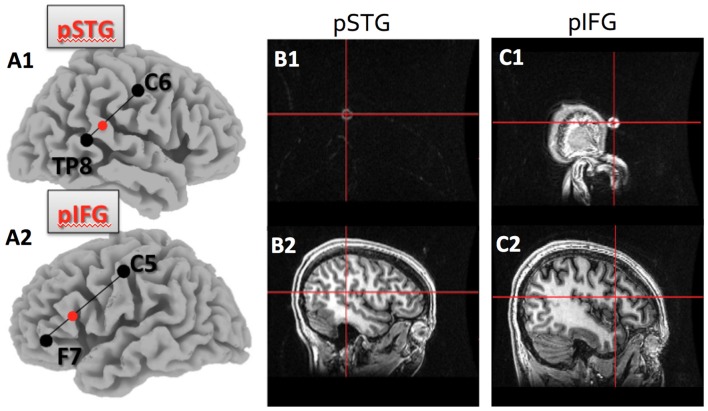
Target location within the posterior superior temporal (pSTG) and posterior inferior frontal gyri (pIFG) as identified by the 10–20 system, and verified using T1 MRI.(A1) shows the pSTG target location as 1/3 of the distance between TP8 and C6 on the right side; (A2)shows the pIFG target location as 1/3 of the distance between F7 and C5 on the left side; (B1) is theMRI marker and crosshair on the skull surface for the pSTG region and (B2) is the same crosshair overthe pSTG target; (C1) is the MRI marker and crosshair on the skull surface for the pIFG; (C2) is the samecrosshair over the pIFG target.
